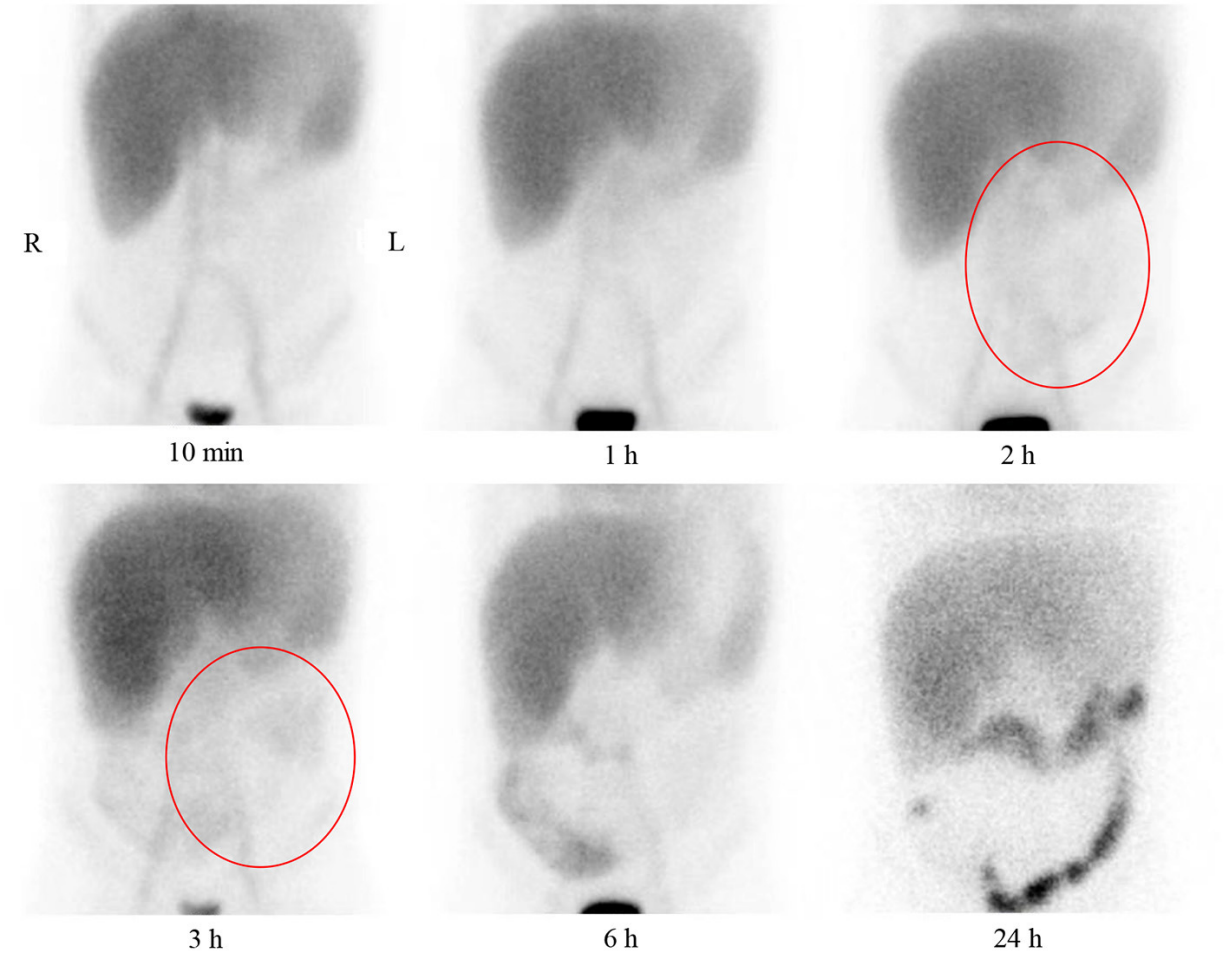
Anterior plane abdominal scintigraphy at 10 min and 1, 2, 3, 6, and 24 h after intravenous administration of technetium-99m-labeled human serum albumin. Tracer accumulation in large vessels, heart blood pool, liver, and kidney is present at 10 min and 1 h. Tracer accumulation in the abdomen is not present at 10 min and 1 h. Tracer accumulation in the lower abdomen is vaguely observed at 2 and 3 h (red circles). A large amount of tracer accumulation is present in the right lower abdomen at 6 h, and the radionuclide shows the bowel. Clear colonic tracer accumulation is present at 24 h.
